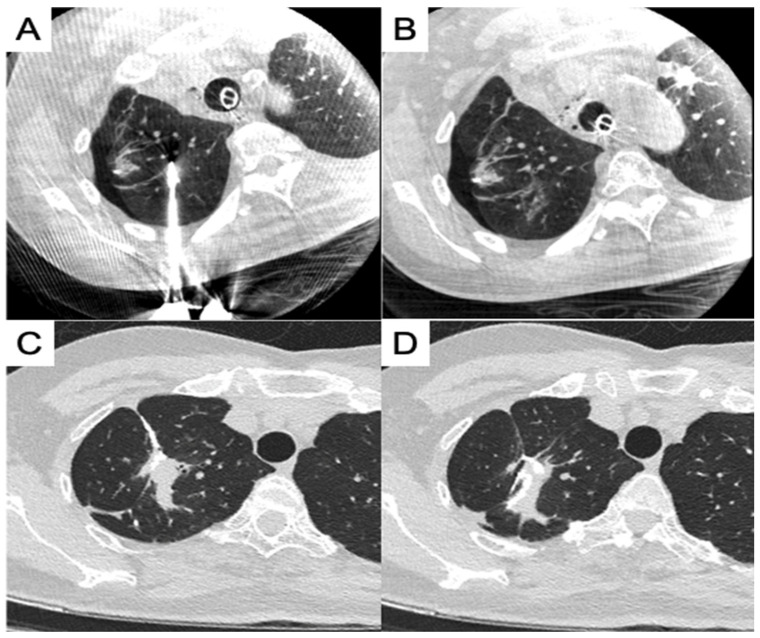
Combined ablation and wedge resection of the same pulmonary lobe. (A) Ablation placement after partial resection; (B) focal ablation zone away from the staple line; (C) post-procedure CT showed a staple line near the ablation zone; (D) post-procedure CT showed a staple line in the ablation zone.
